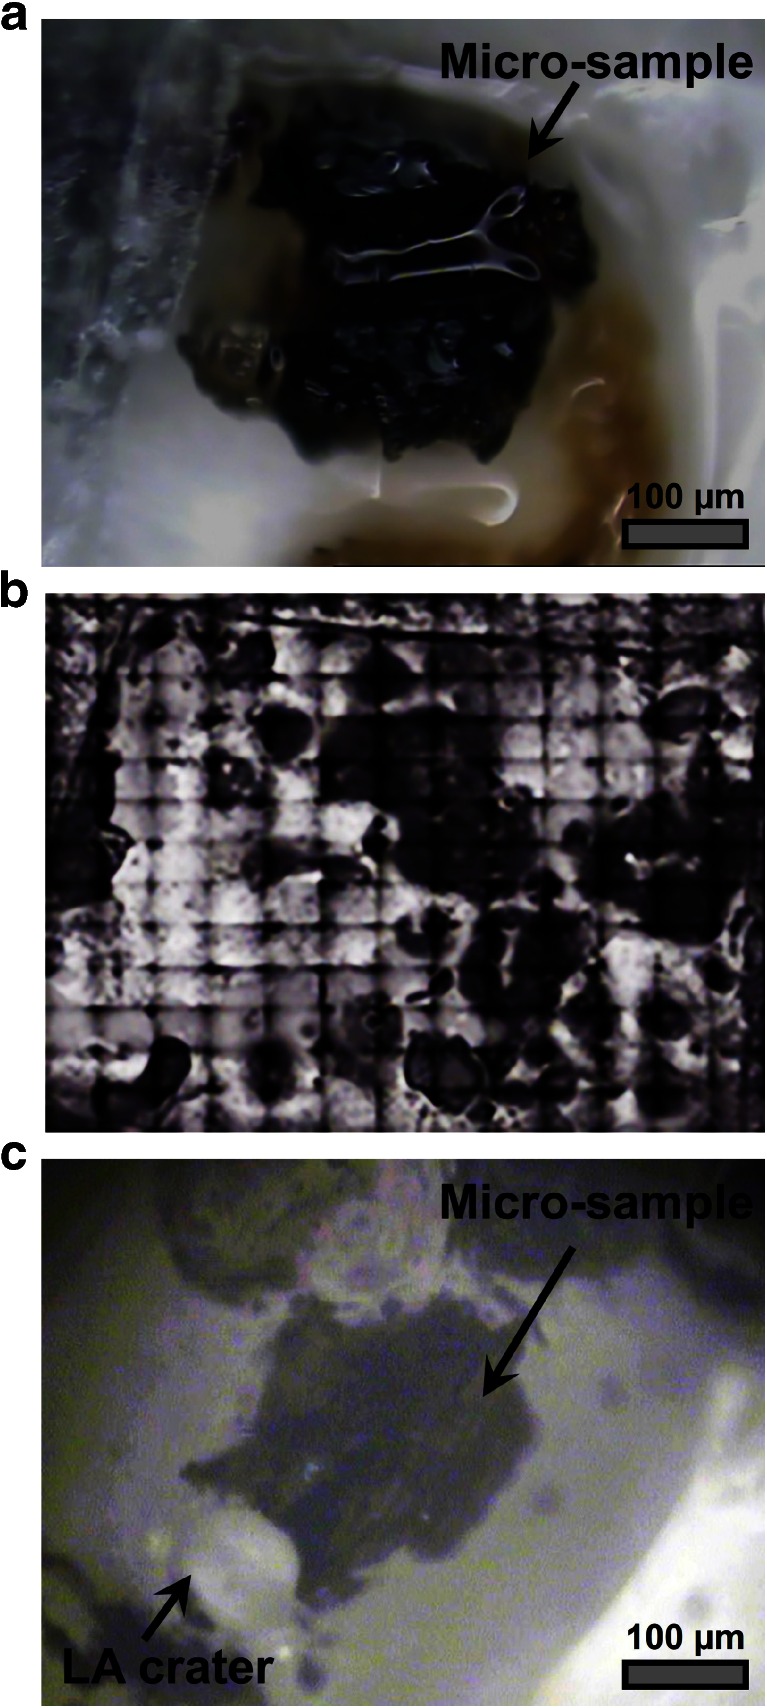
Depiction of micro-samples (radioactive fuel particle clusters) that were collected in the vicinity of the accidental Chernobyl reactor: (A) typical micro-sample embedded in a transparent membrane after pre-selection by nuclear track radiography, (B) LA sample map overview of a transparent membrane (∼507 mm^2^) containing several micro-samples (*dark spots*) and (C) typical micro-sample after performing one static point ablation
